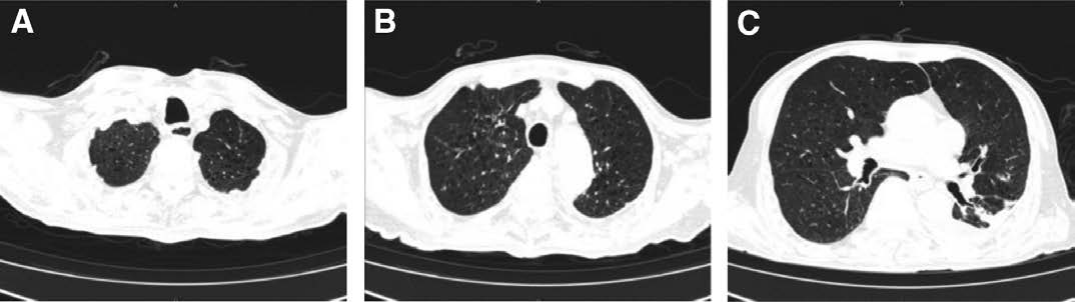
Thoracic CT scan following the removal of the pleural effusion catheter and tracheostomy tube from the patient. (A–C) The images indicated significant improvement in the patient’s pleural effusion and pulmonary infection. CT = computed tomography.
